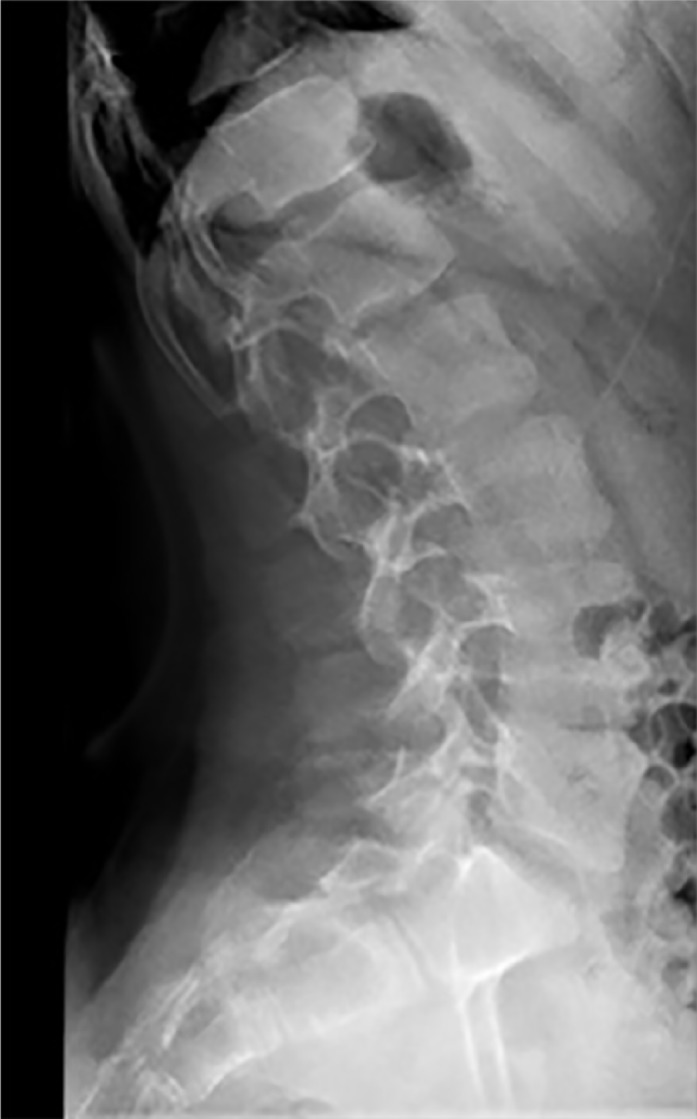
Lateral spine radiograph in a 10-year-old child with Klinefelter syndrome showed exaggerated lumbar lordosis, mild platyspondyly of T11-12, anterior end-plate irregularity of T12, scalloping of the posterior end plate of L3.
